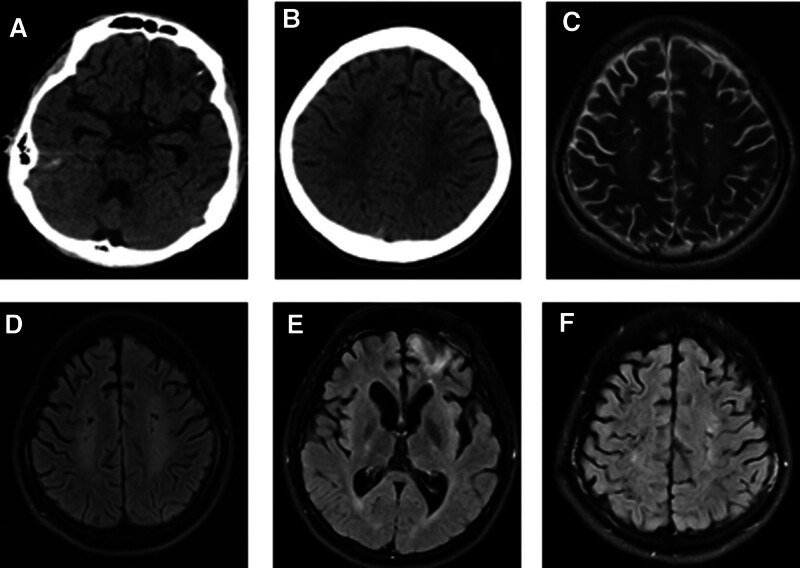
One month following the trauma, a CT scan revealed left frontal malacia (A), along with bilateral centrum semiovale hypodensity (B). Concurrently, the skull MRI indicated high T2WI signals and low T2 FLAIR signals (C and D), suggesting the presence of diffuse axonal injury. On the 6-mo magnetic resonance imaging, there was evidence of left frontal malacia, mild cerebral atrophy (E), and bilateral centrum semiovale hyperdensity (F). CT = computed tomography, MRI = magnetic resonance imaging.
